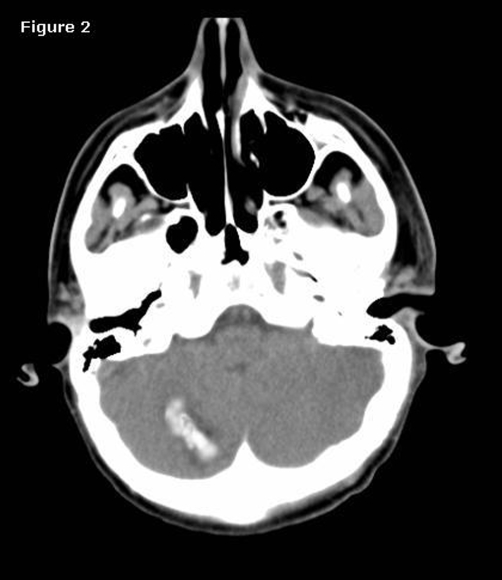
Immediate postoperative CT scans show acute hemorrhage in the right cerebellum fulia.
